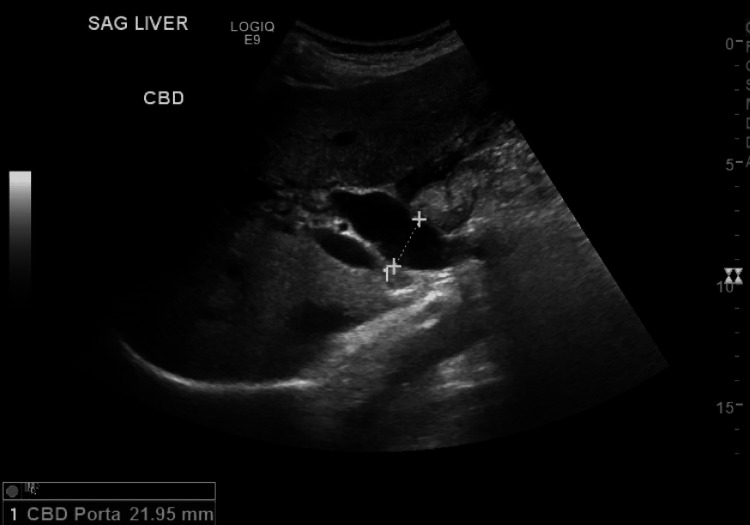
Ultrasound of the abdomen and pelvis revealing cholelithiasis and acute cholecystitis Sagittal view of the liver with measurements of the common bile duct, measuring approximately 22 mm.
